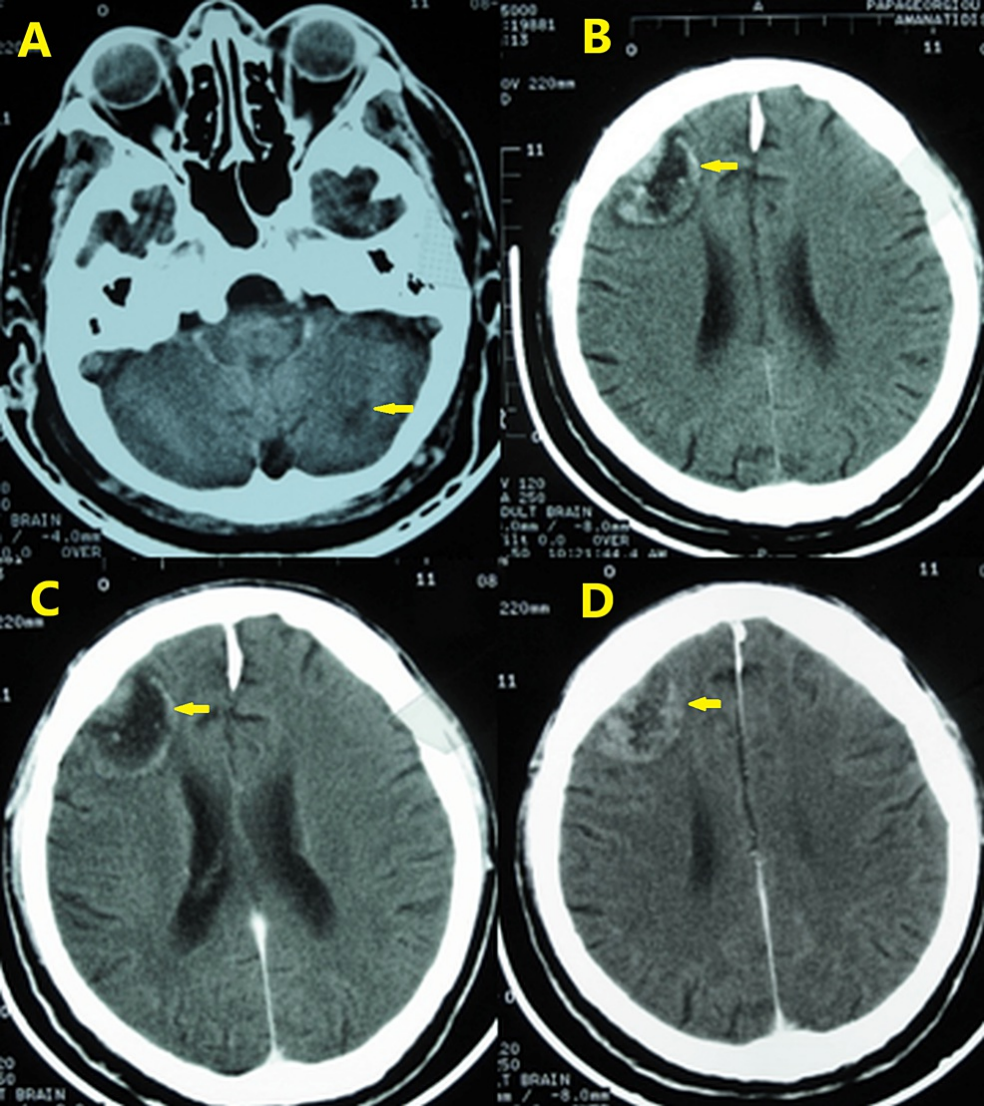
Brain computed tomography scan on July 8, 2008. Axial CT images plus contrast medium. In the left cerebellar hemisphere, there is a small low-density lesion without enhancement post-contrast medium and no perifocal edema or mass effect (A). Reduction in the size of the right frontal lobe lesion from 5.5 cm × 6.2 cm to 4 cm × 2.7 cm with no perifocal edema or mass effect on the right ventricle (B-D). No left lesions in the left frontal lobe and the right temporal lobe.
